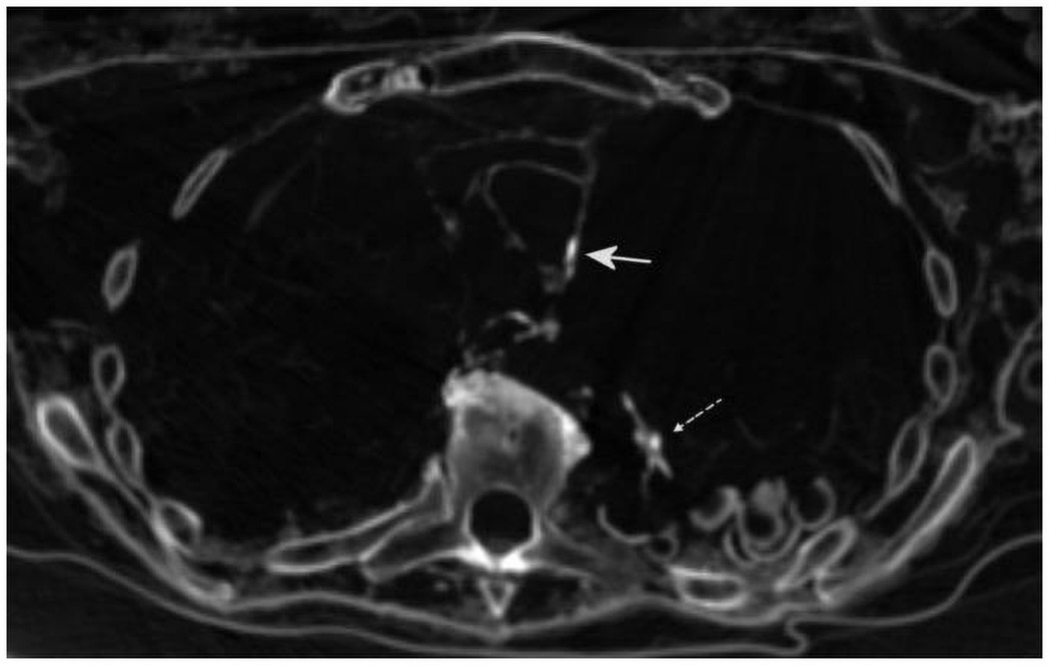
CT scan cross section through the thorax showing focal calcifications of the vessel wall (full arrow); note the peripheral pulmonary calcifications (dotted arrow) that occurs occasionally in this cut of the left lung. Additionally, the shell-like irregular objects are clearly visible.
